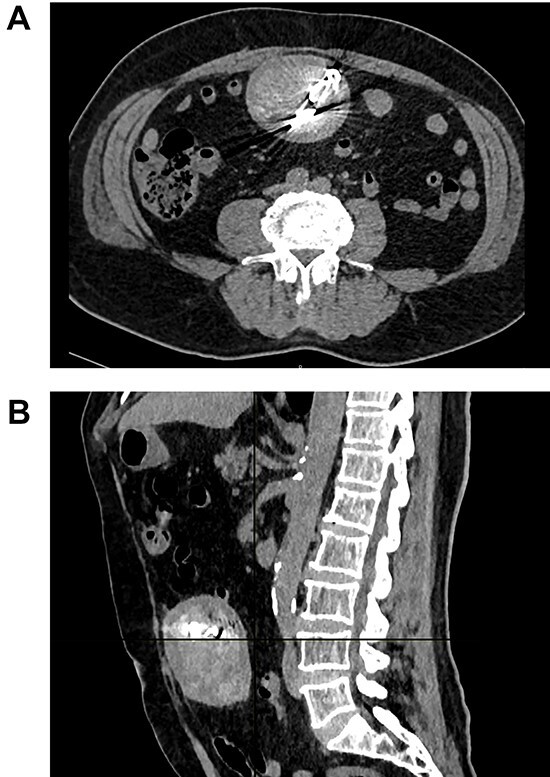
(A) CT the mass is seen in the abdomen with radiopaque material on its center. (B) CT the mass attached to the bowel.
